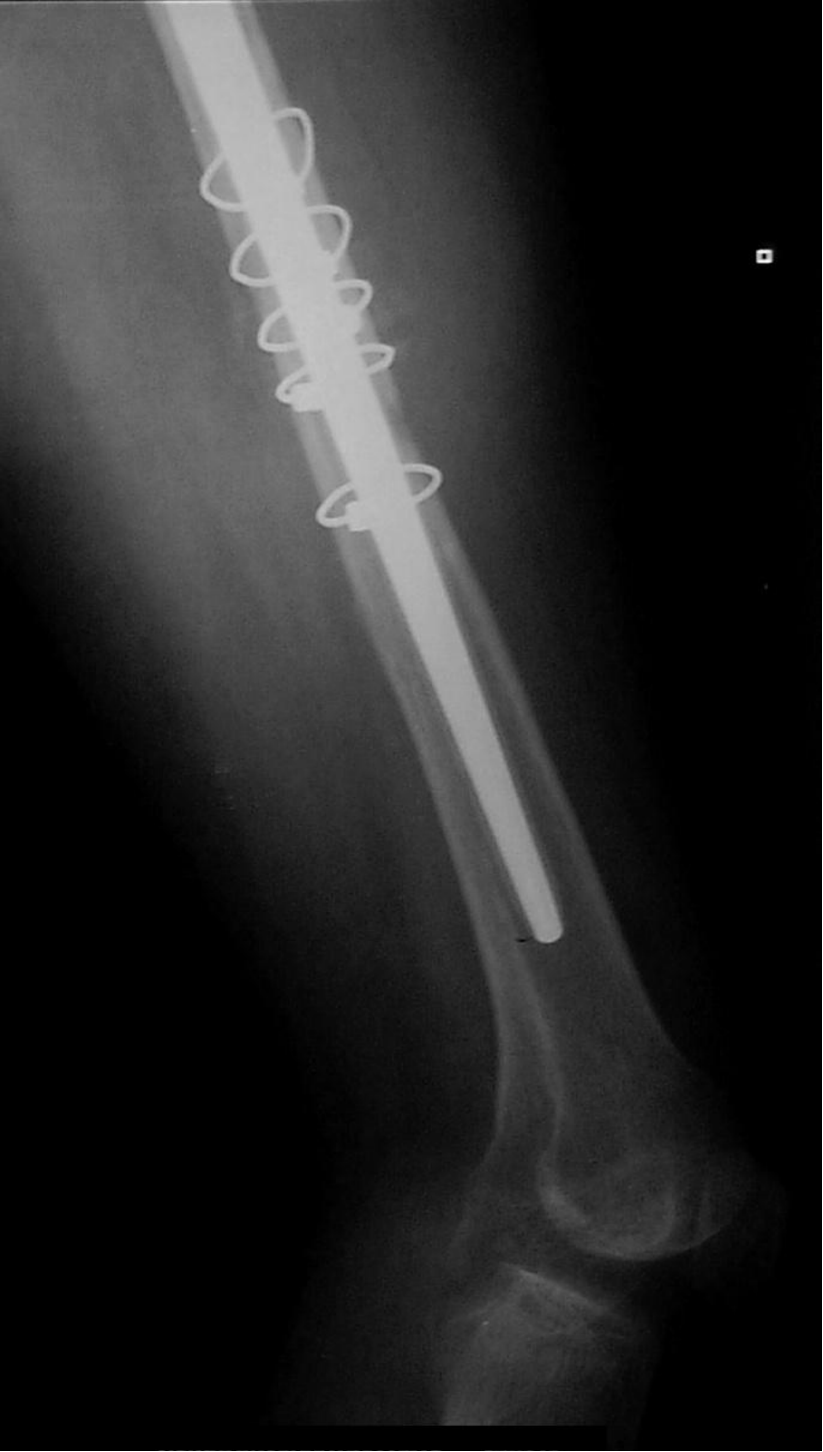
Lateral X-ray at three postoperative months. The fracture has healed.
